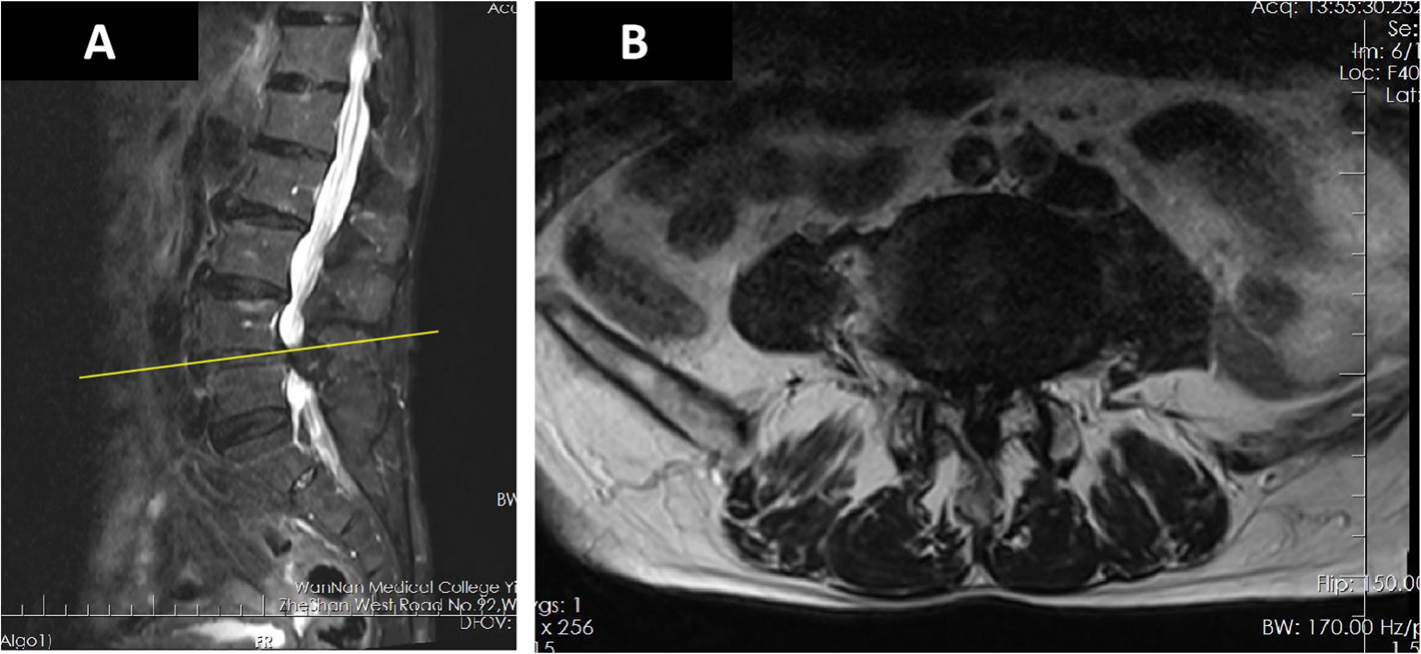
Sagittal (a) and transverse (b) fat-suppressed magnetic resonance imaging of the lumbar spine indicated that the spinal canal was extremely narrow
